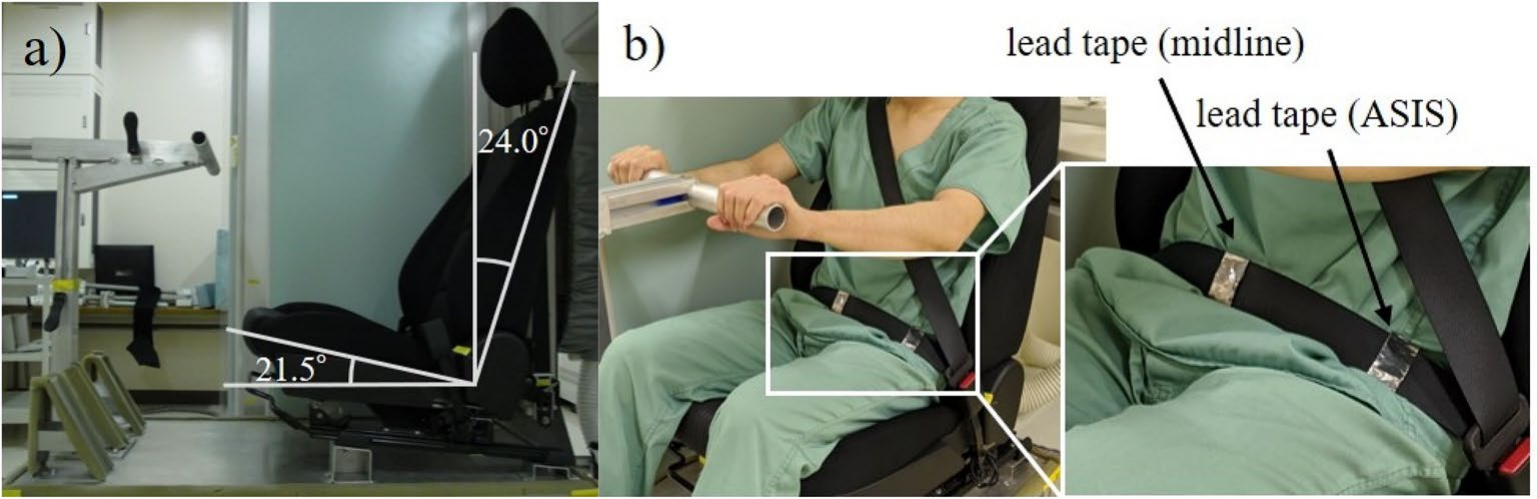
X-ray table using Mazda 3 car seat. (a) The back of the seat was fixed at 24 degrees, and the seat surface at 21.5 degrees. The handle that imitates the steering wheel, and the pedals, were placed in the same positions as in the actual vehicle. (b) The midline and left ASIS equivalent positions of the lap belt were marked by wrapping 3 M™ lead foil tape 420 (25.4 mm × 32.9 mm) around each position so that they could be identified radiographically. In each case, the first author palpated and confirmed the center of the abdomen and the left ASIS, and positioned the markings.
